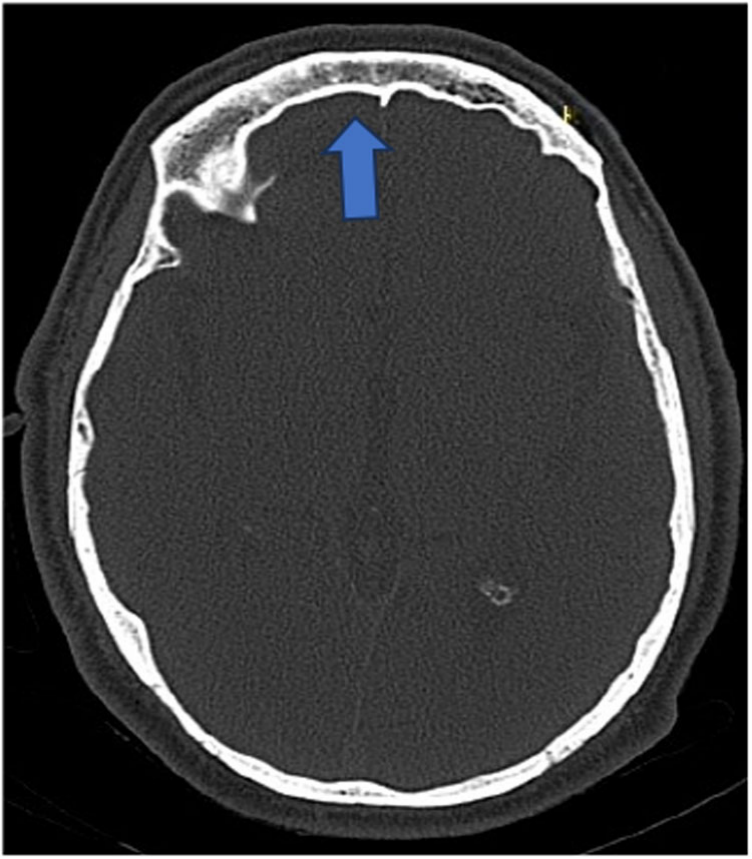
CT axial image shows aplasia of frontal sinus.
